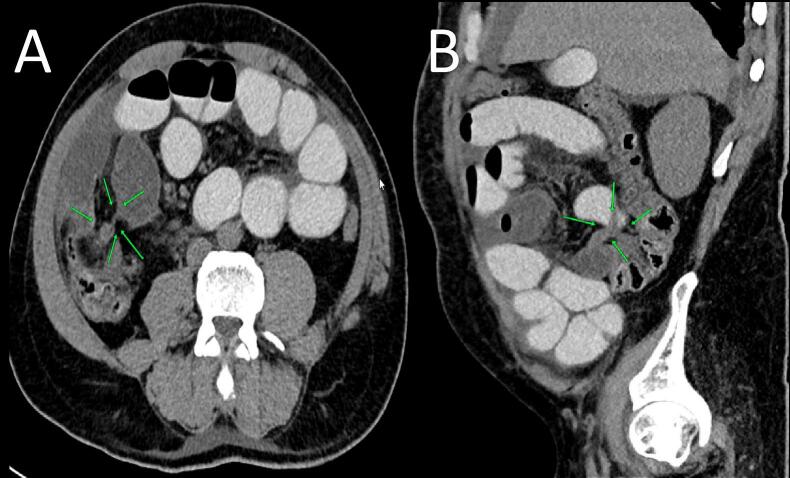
Axial (A) and Sagittal (B) CT scan showing a small segment of terminal ileal narrowing and multiple dilated small bowel loops consistent with small bowel obstruction.
